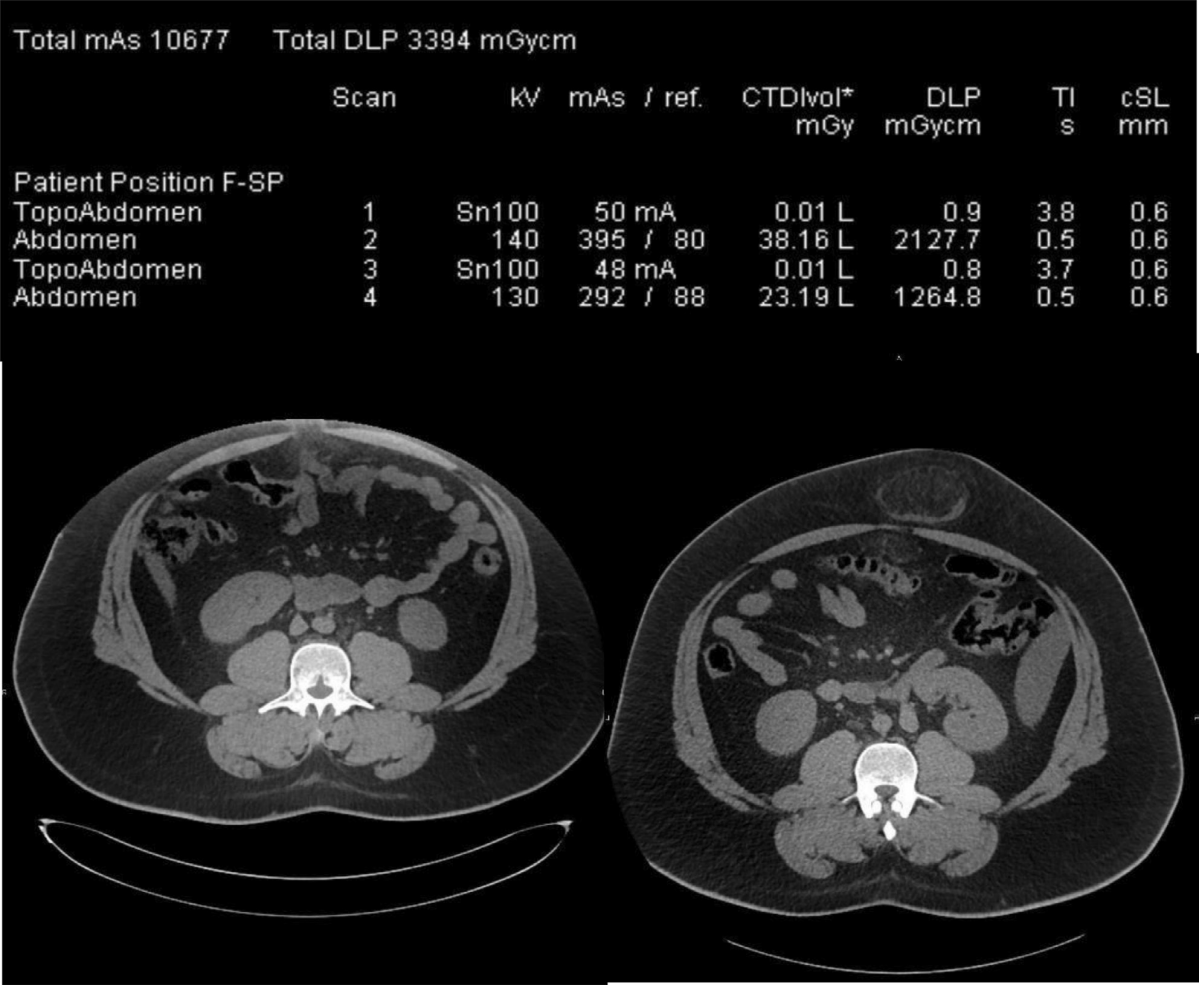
Radiation dose report and CT slices obtained at the level of the umbilic in a 24 year-old patient. The left CT slice is off-centered and the herniation is not visible whereby the right CT slice of correctly centered and the herniation is visible. The two first exposures on the dose report correspond to the left image whereby the two last lines correspond to the right image including the umbilical herniation.
